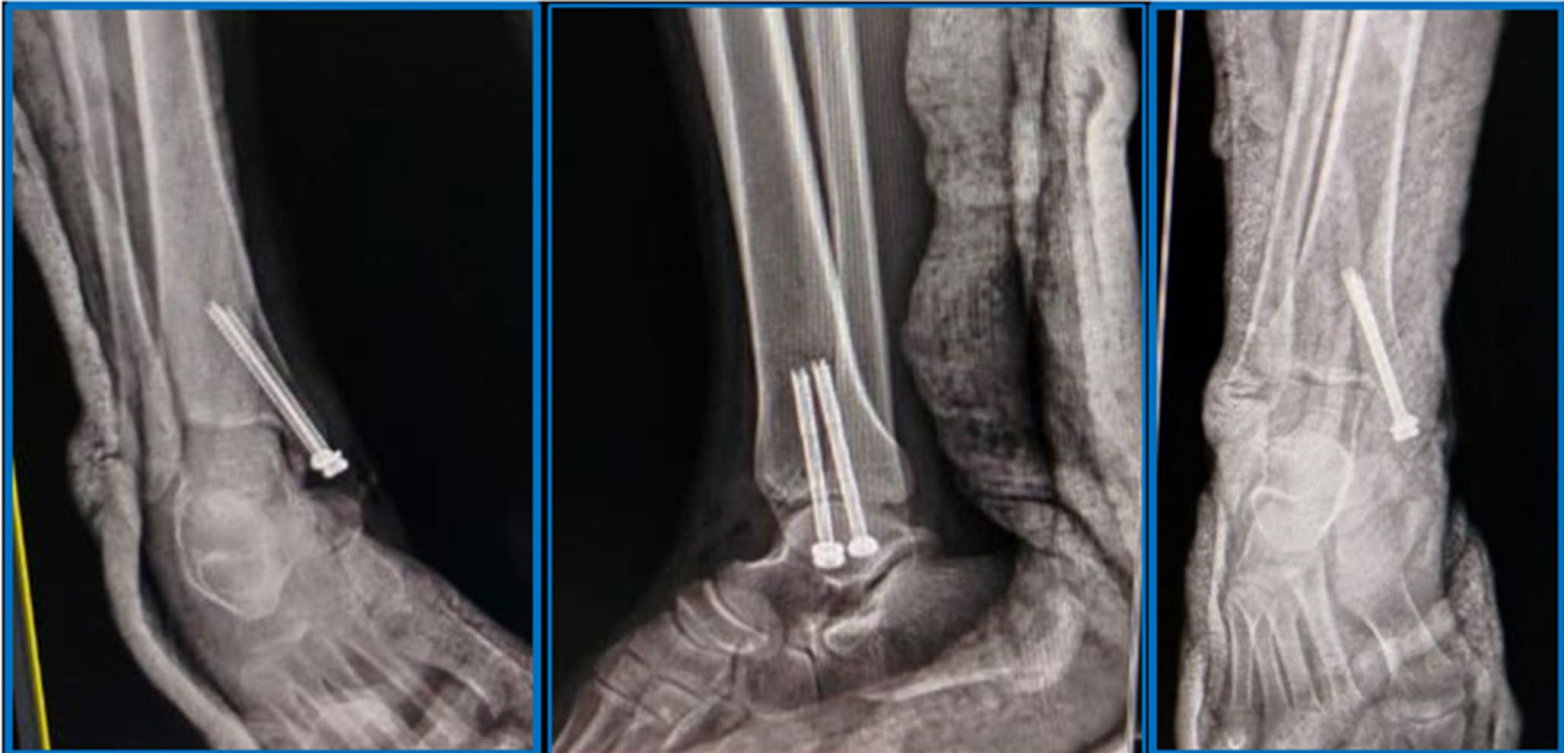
Surgical technique of CRPF (continued): post-operative radiographs
